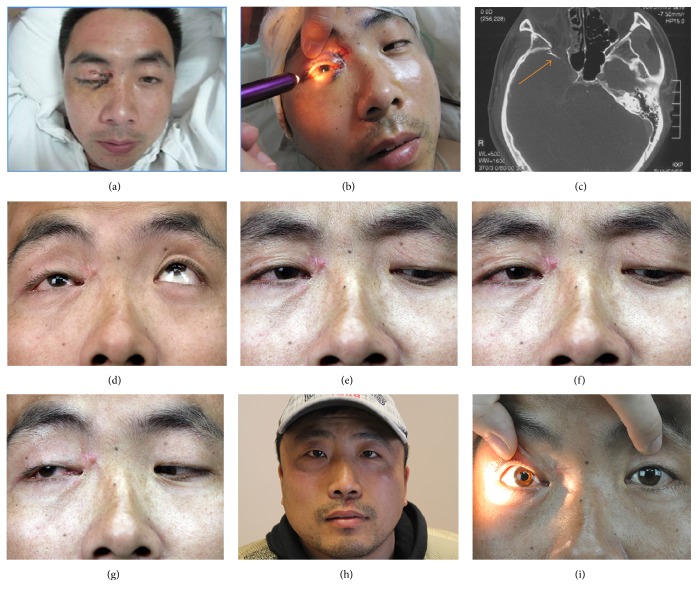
(a) Preoperative physical examination showed ptosis of right eye and contusion of the surrounding soft tissues; (b) dilated pupil of the right eye before surgery; (c) presurgical CT demonstrated fractures of right orbital apex (the orange arrow means there were obvious fractures in the orbital apex region); (d–g) the eye movement was significantly improved with slightly restriction at 3 months after the operation.
